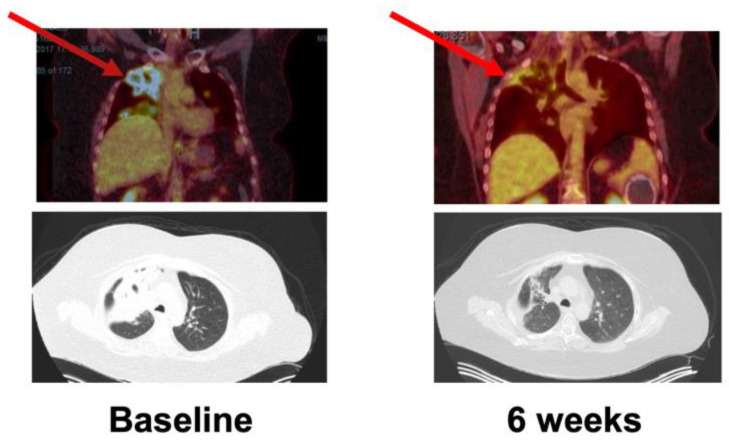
Partial response in patient with a head and neck squamous cancer and loss of function NOTCH1T1997M mutation. A partial response in a patient with metastatic HNSCC and NOTCH1T1997M loss of function mutation demonstrated on PET/CT and obtained after 6 weeks of therapy compared to baseline. The patient received the intermittent dosing schedule A (200 mg).
